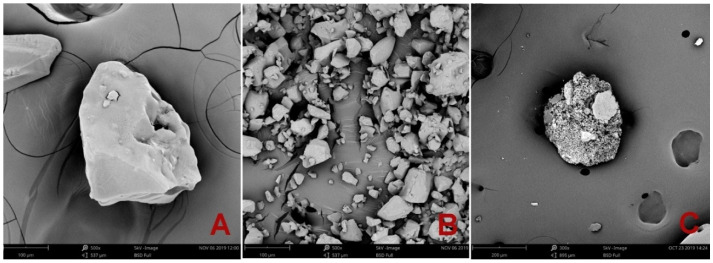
SEM images of (A)—Creasorb SIS; (B)—Cabloc CT; (C)—Hydropam.
